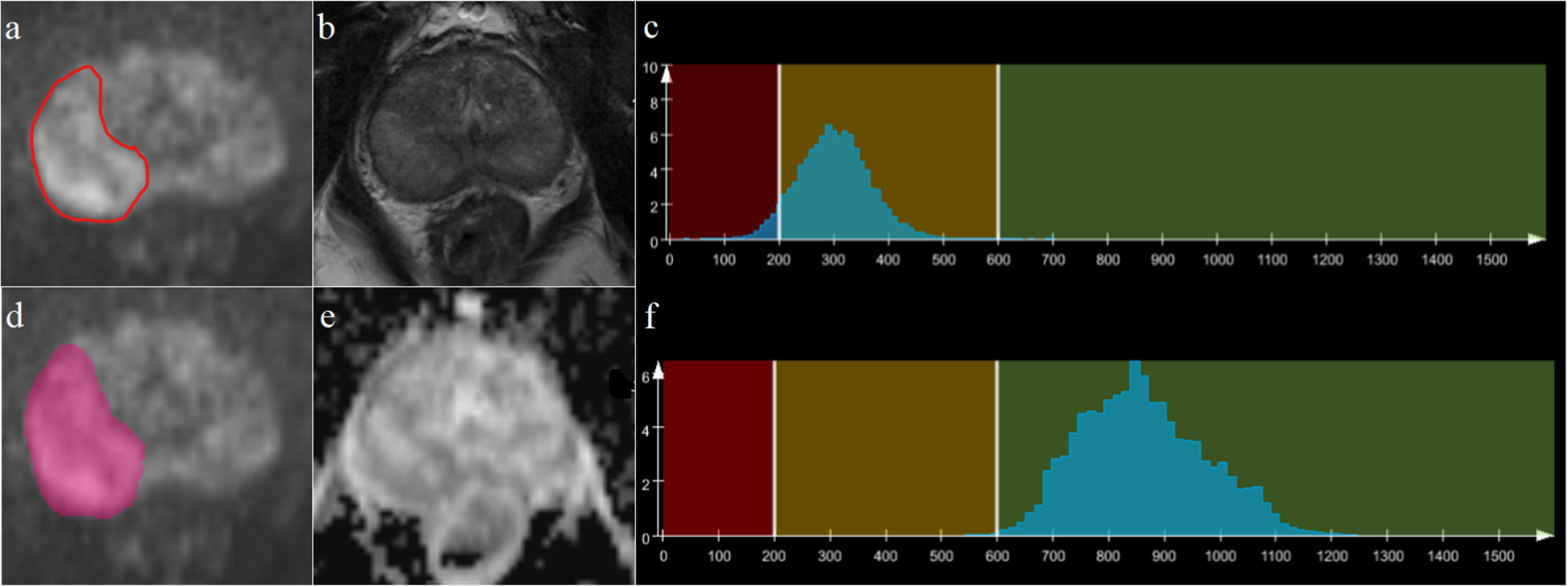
Representative images of a 63-year-old-male patient with benign prostatic hypertrophy (a-f). Segmentation of the lesion is shown on diffusion-weighted imaging (DWI) images with a *b* = 1500 s/mm^2^ (a, d). Histograms (c, f) of T2weighted (T2W) images (b) and apparent diffusion coefficient (ADC) maps (e) were inline-generated
